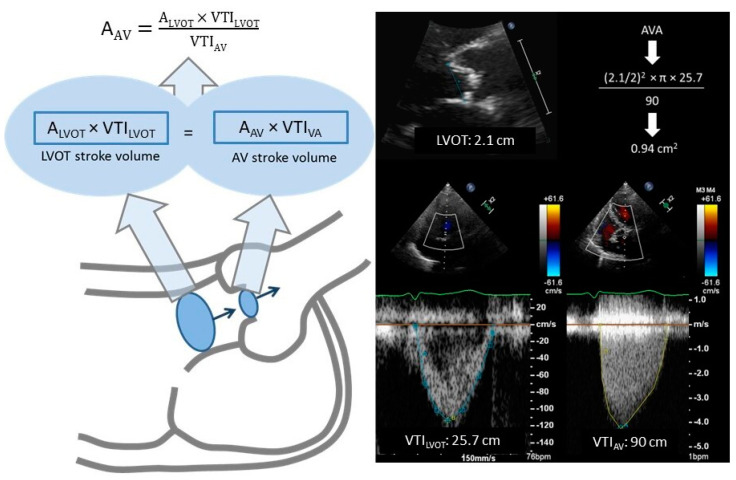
Continuity equation. A_AV_: aortic valve area, A_LVOT_: LVOT area, estimated via the circle area formula: [(Diameter/2)^2^ × π], VTI_AV_: velocity time integral at the aortic valve orifice (calculated with CWD aligning the ultrasound beam parallel to the systolic blood flow through the aortic valve), VTI_LVOT_: velocity time integral at LVOT (assessed with PWD).
